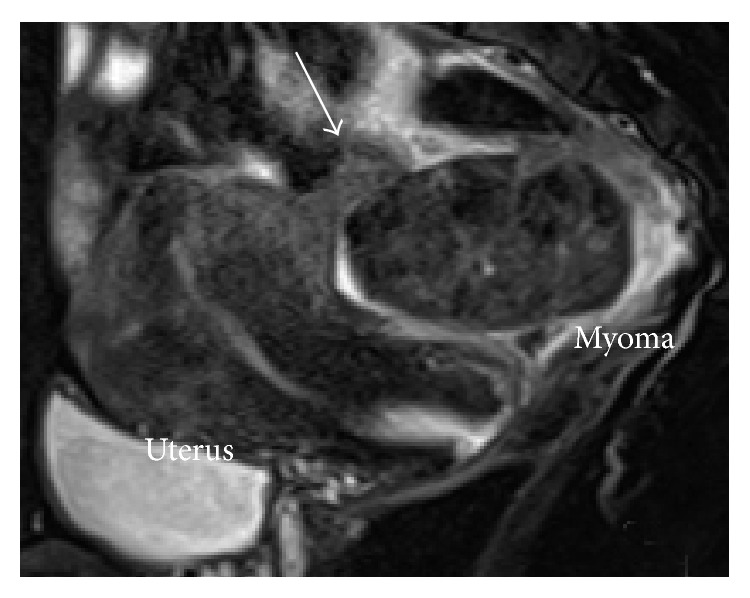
Sagittal T2-weighted MRI showing a torted pedicle of the subserosal myoma (arrow).
